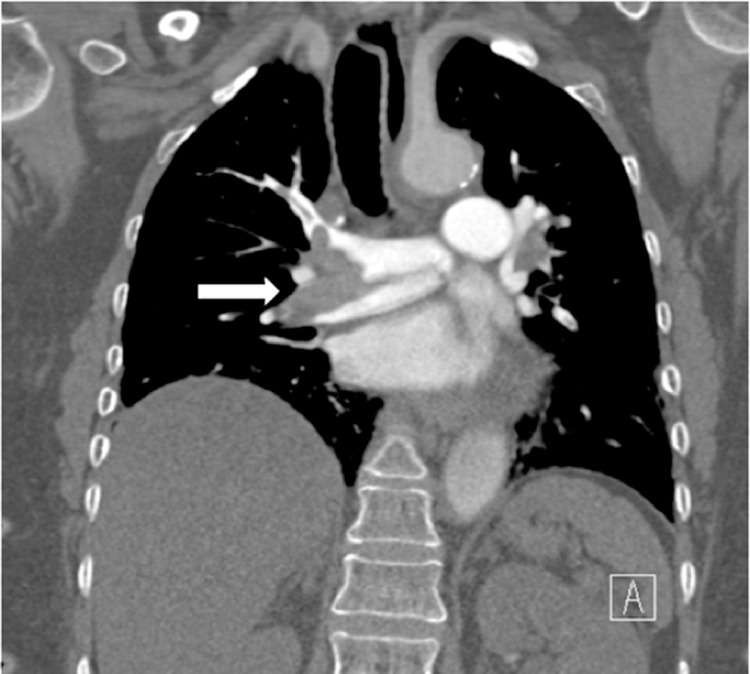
(Case 2). Chest CT demonstrated acute pulmonary embolism with saddle embolus in the right pulmonary artery (arrow).
